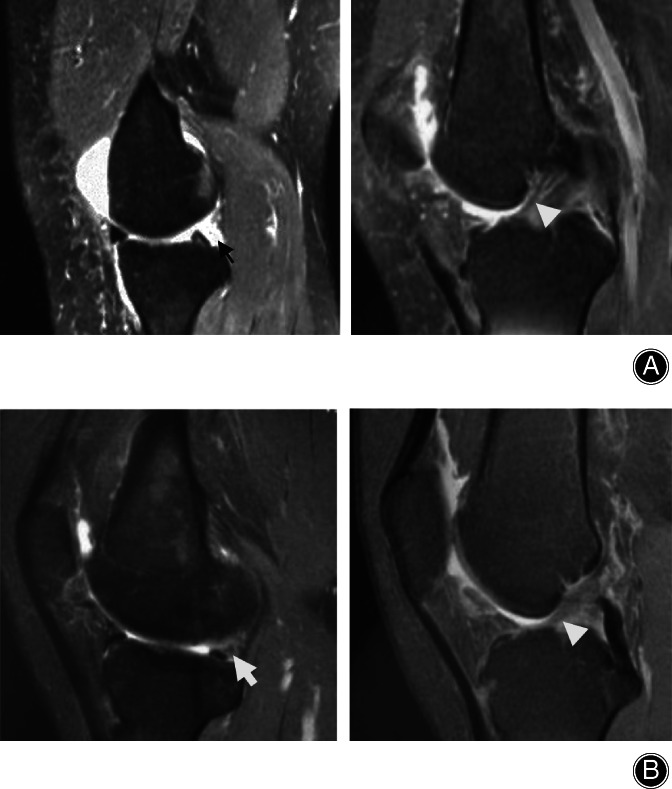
A 36‐year‐old female patient suffered from posteromedial left knee pain without obvious anterior cruicate ligament (ACL) rupture. (A) Preoperative fat suppression (FS) T2 MRI sagittal image shows isolated ramp lesion of the posterior horn of medial meniscus (PHMM) (black arrow) with intact but degenerative ACL integrity (white triangle), manifesting as high signal fluid between the PHMM and the posterior capsule. (B) After 6 months, postoperative FS T2 MRI sagittal images show healing of the ramp lesion (white arrow), manifesting as low signal meniscocapsular attachment tissue of the PHMM.
